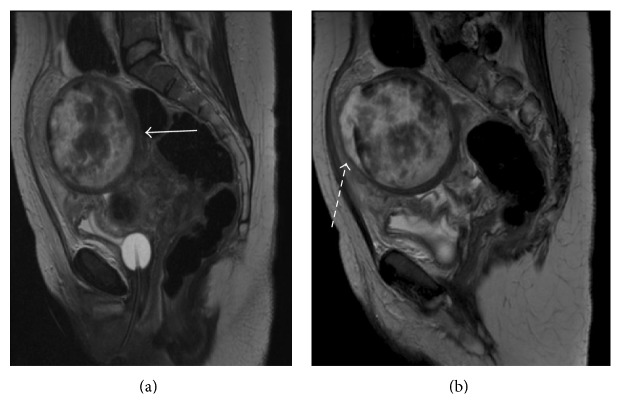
MRI of the PELVIS. (a) Necrotic 8.2 cm subserosal fibroid [arrow]. (b) Necrotic 8.2 cm subserosal fibroid with an encapsulated collection lying anterior to the fibroid suggesting rupture [dashed arrow].
